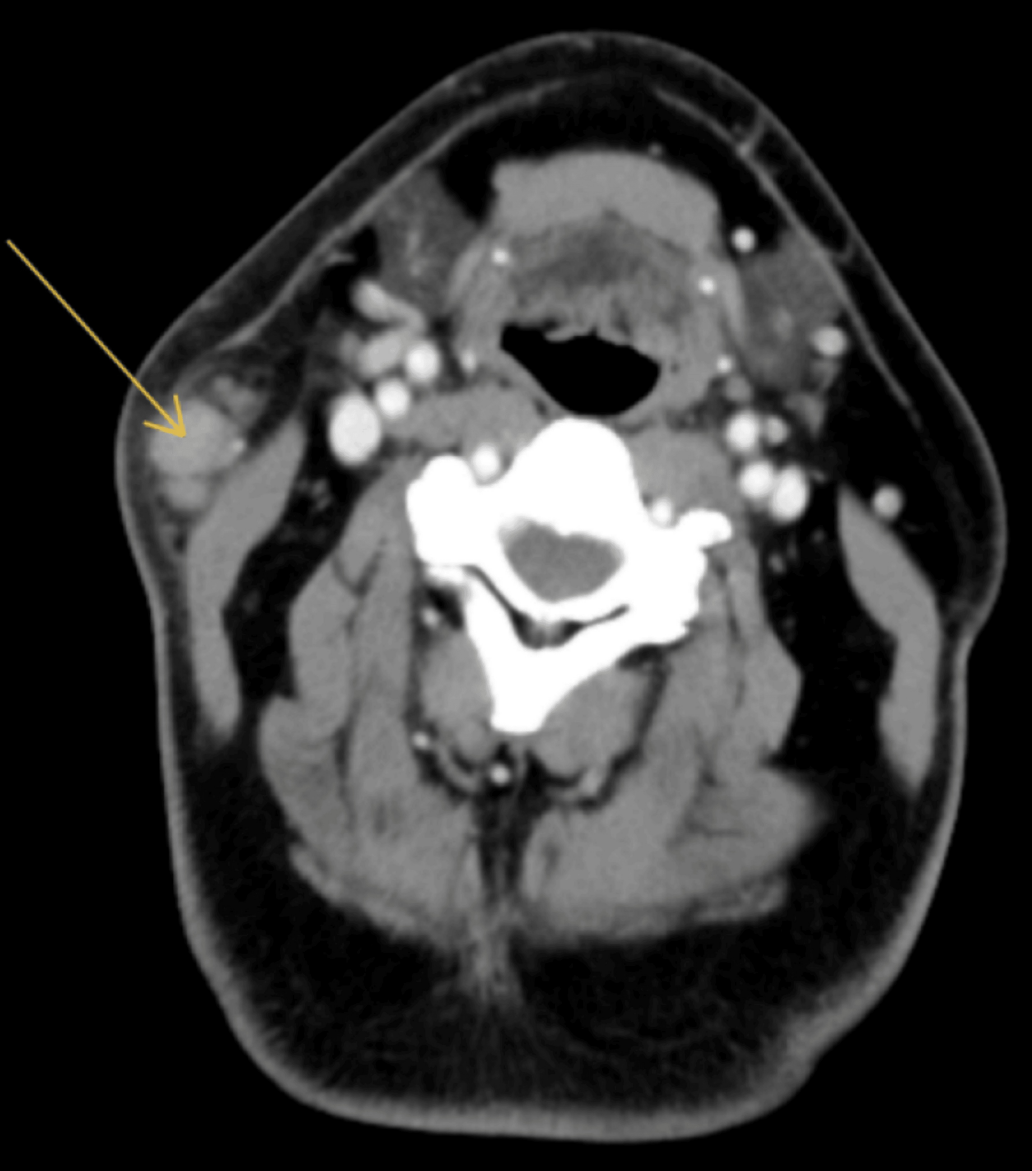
Axial CT denoting enlarged cervical lymph node with surrounding soft tissue stranding
